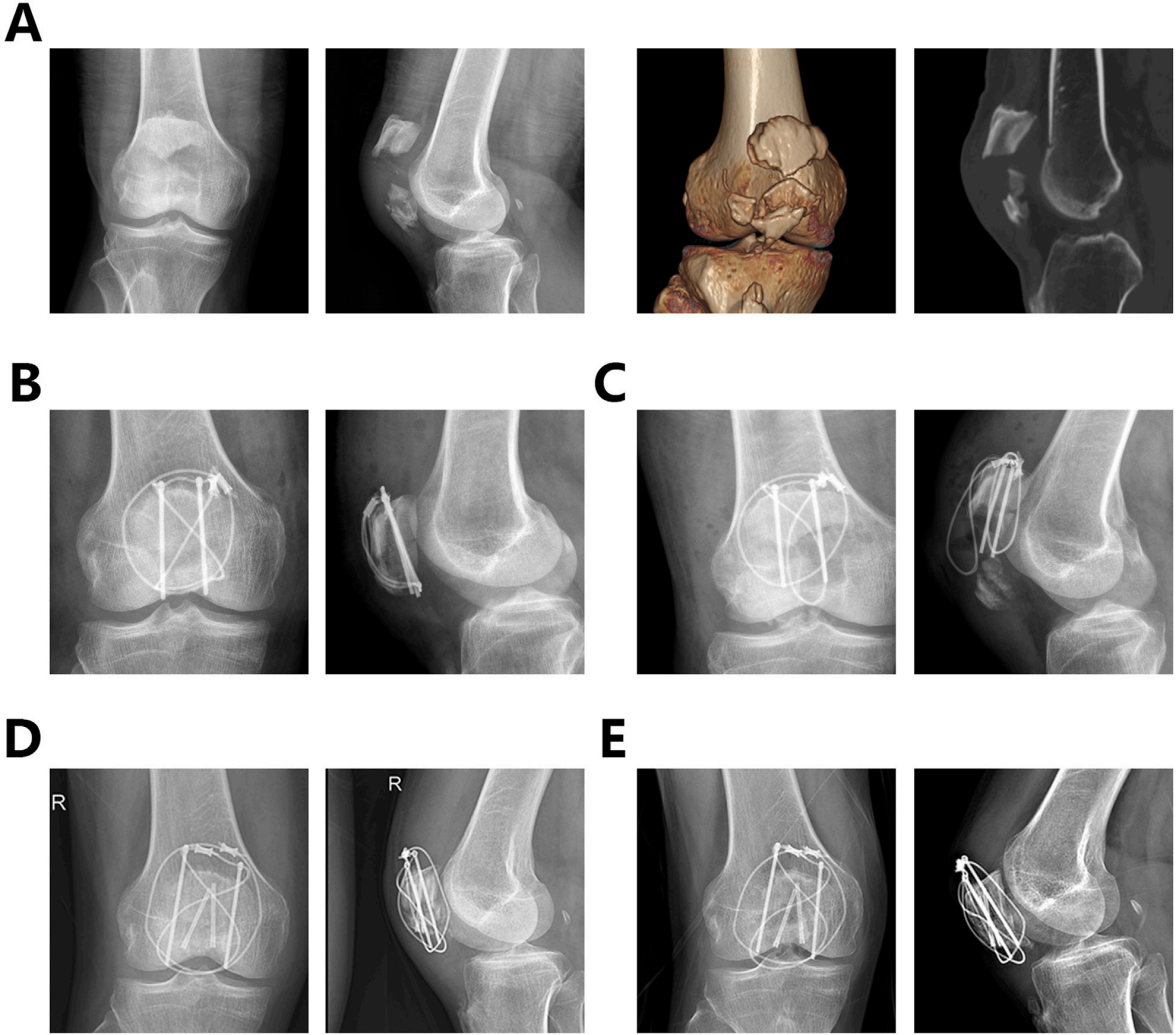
A 65-year-old male patient underwent internal fixation failure and revision surgery after being treated with a single tension band of internal fixation (A). Preoperative x-rays and CT images (B). Postoperative frontal and lateral views of the knee after the first surgery (C). Postoperative frontal and lateral views of the knee at 2 months postoperatively showing failure of the internal fixation and displacement of the fracture (D) Postoperative frontal and lateral views of the knee after the second surgery (E). Postoperative frontal and lateral views of the knee at one year postoperatively after the second surgery.
